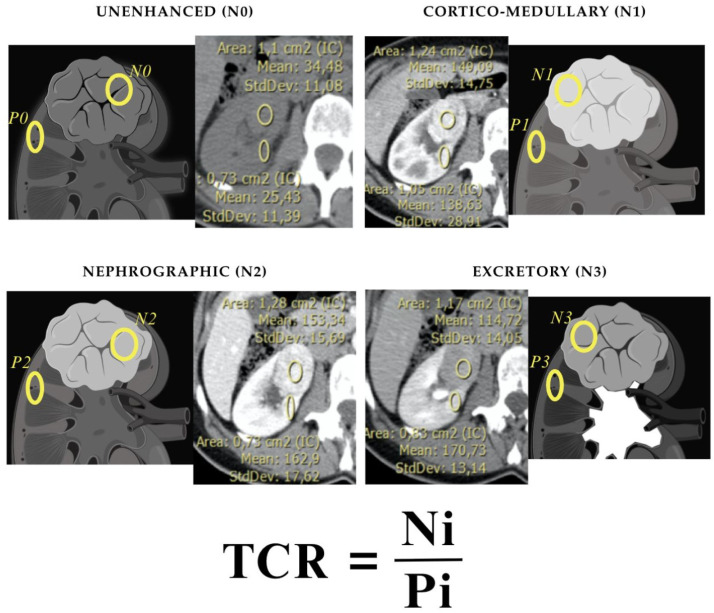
Tumor-to-cortex ratio (TCR), calculated as the ratio between lesion attenuation in each CT phase (Ni) and normal cortical attenuation in the same phase (Pi). Images created with BioRender.com.
